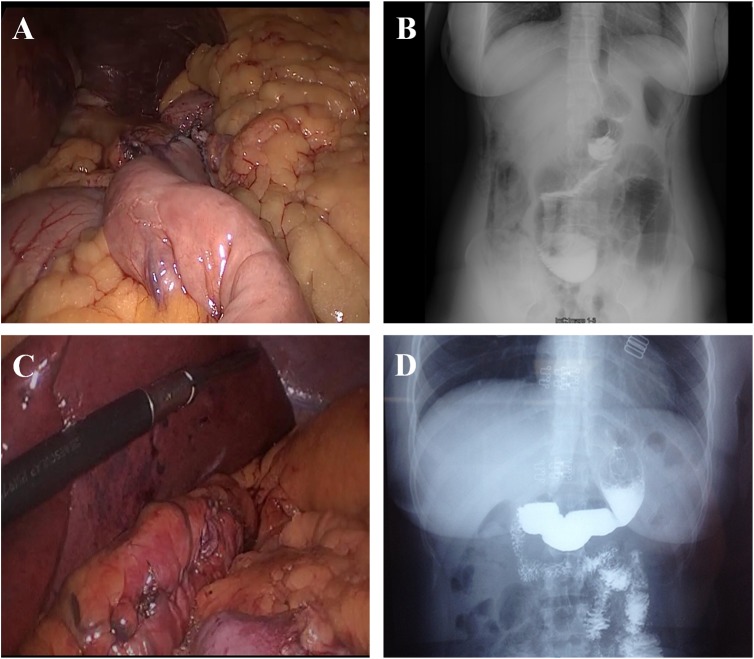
Bariatric surgery for treating obesity A. Intra-operative photograph of the LRYGB procedure. B. Postoperative upper gastrointestinal barium X-ray radiography after LRYGB. C. Intra-operative photograph of the LSG procedure. D. Postoperative upper gastrointestinal barium X-ray radiography after LSG.
